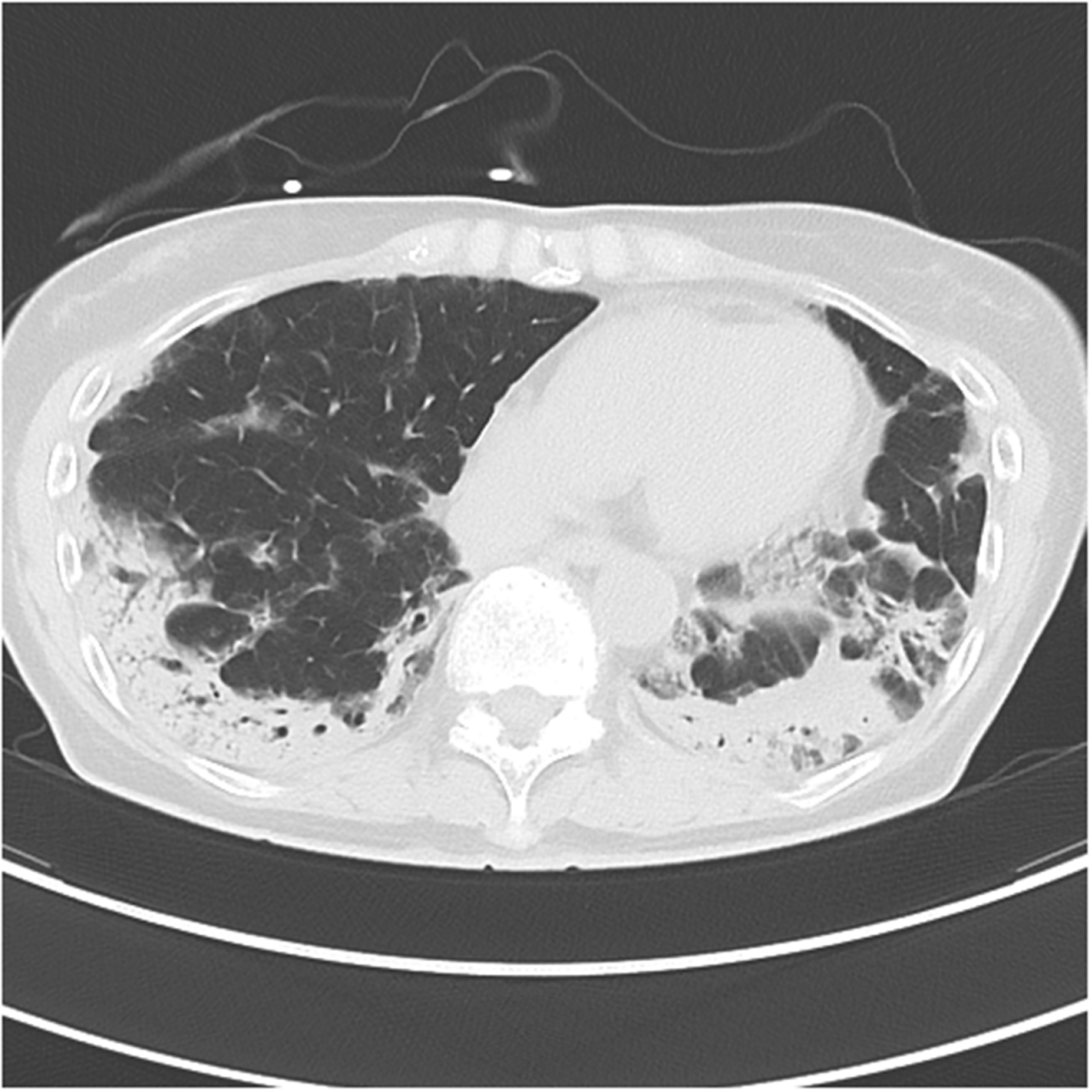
Initial chest computed tomography (CT) showed bilateral and peripheral predominant consolidation and air bronchogram
